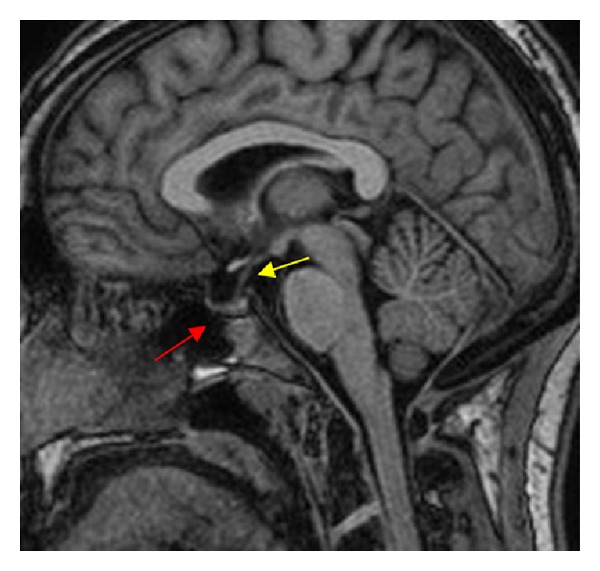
Postoperative sagittal T1 MRI of brain without contrast shows resolution of the prior areas of hyperintensity within the sella turcica (red arrow). The pituitary stalk is now visible (yellow arrow).
